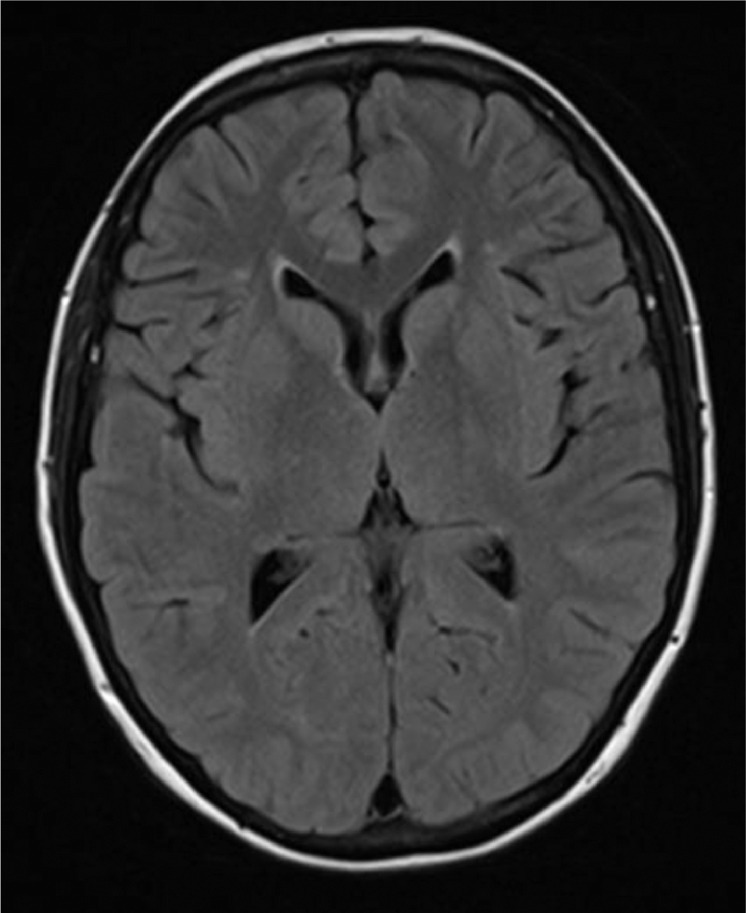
Follow-up axial FLAIR MRI brain demonstrating interval resolution of the white matter signal abnormality.
